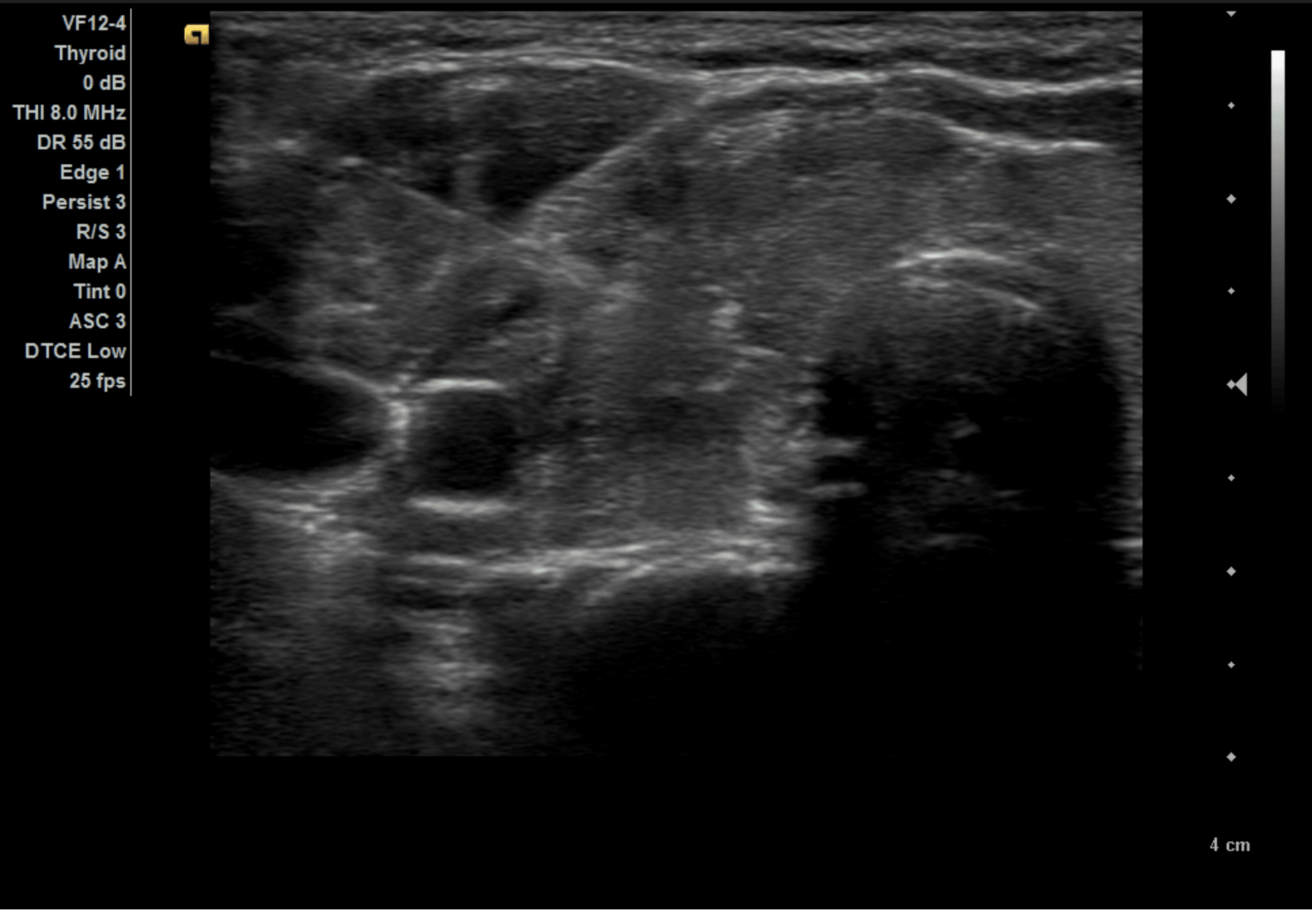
An ultrasonographic view of the right thyroid lobe demonstrates a passed needle through an ill-defined hypoechoic area.
